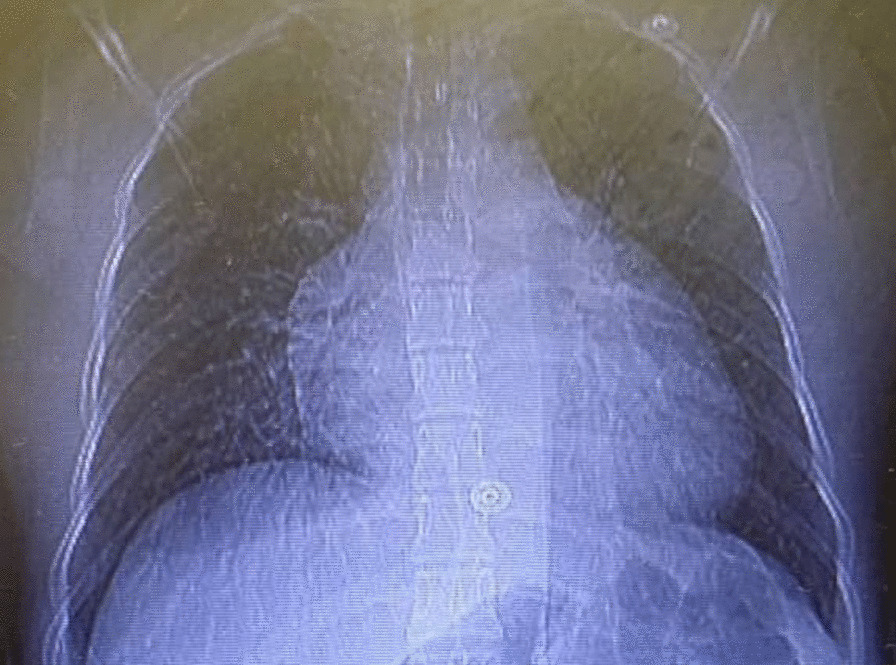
Chest X-ray showing improved COVID-19 infection and no pulmonary edema with cardiomegaly on 12th day of admission
